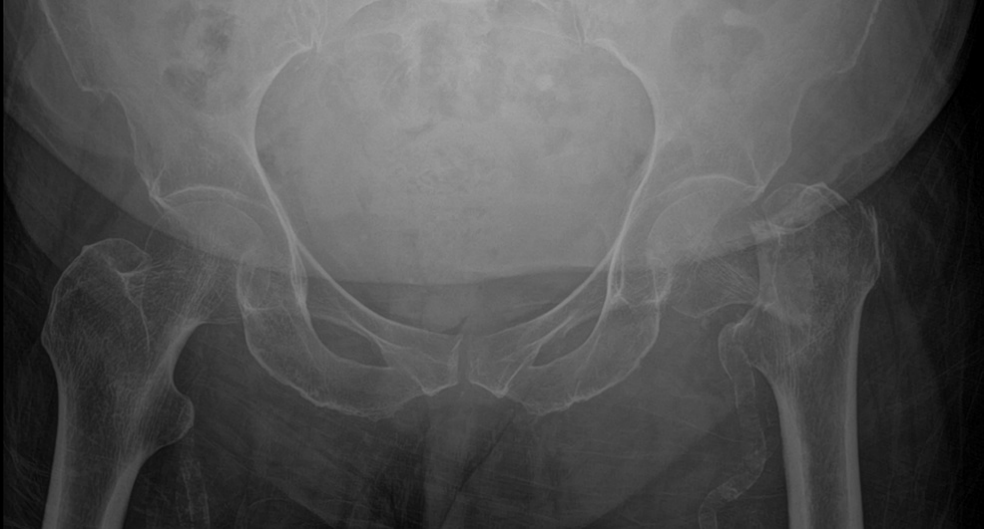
Pre-operative radiograph of the only patient in the study who subsequently had a prosthetic dislocation
